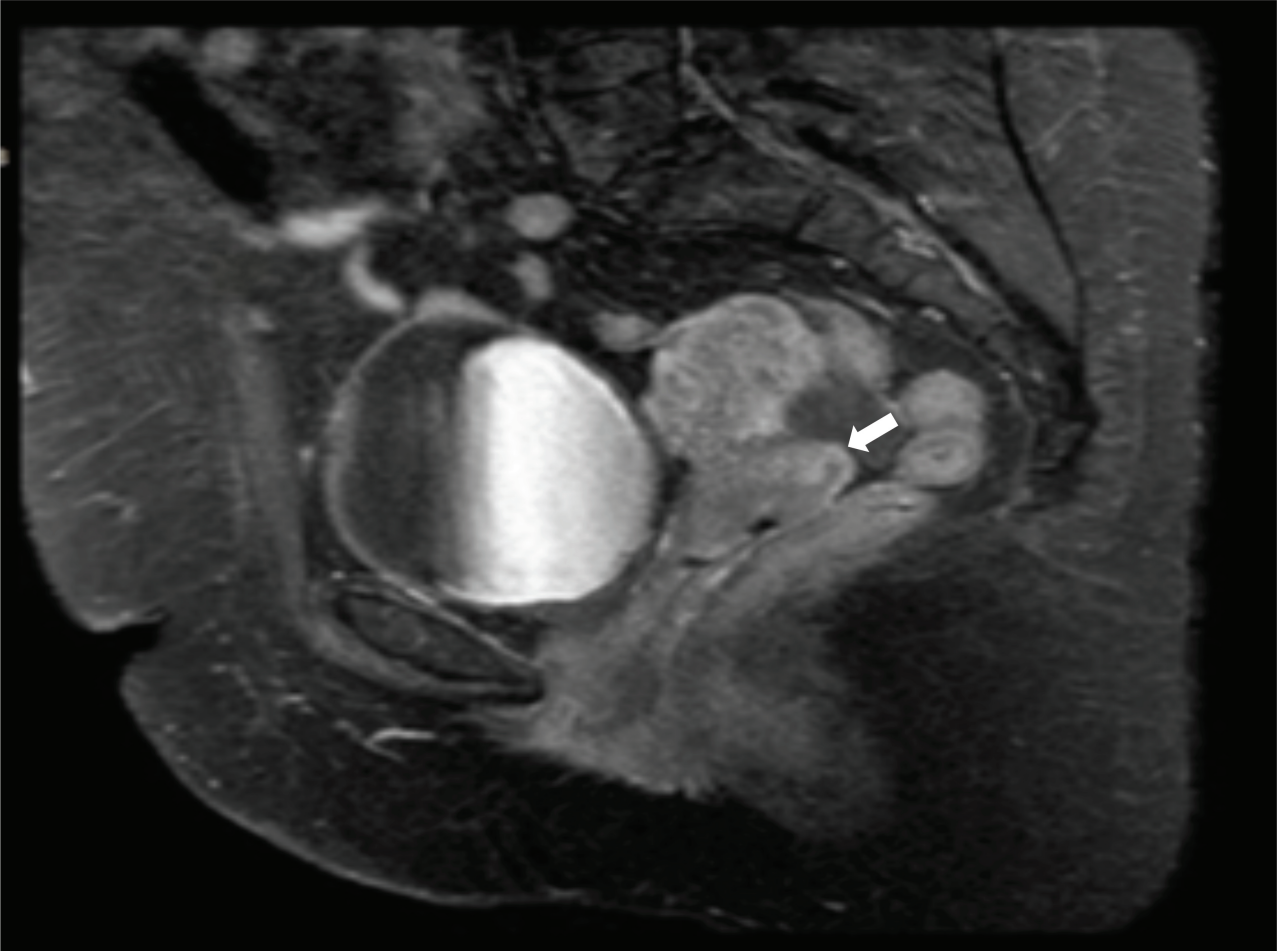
Contrast MRI of the pelvis. Sagittal view. Thickening of 2 × 2 cm at the level of the uterine cervix, predominance of the posterior lip that shows diffusion restriction and enhances with the contrast substance (→).
